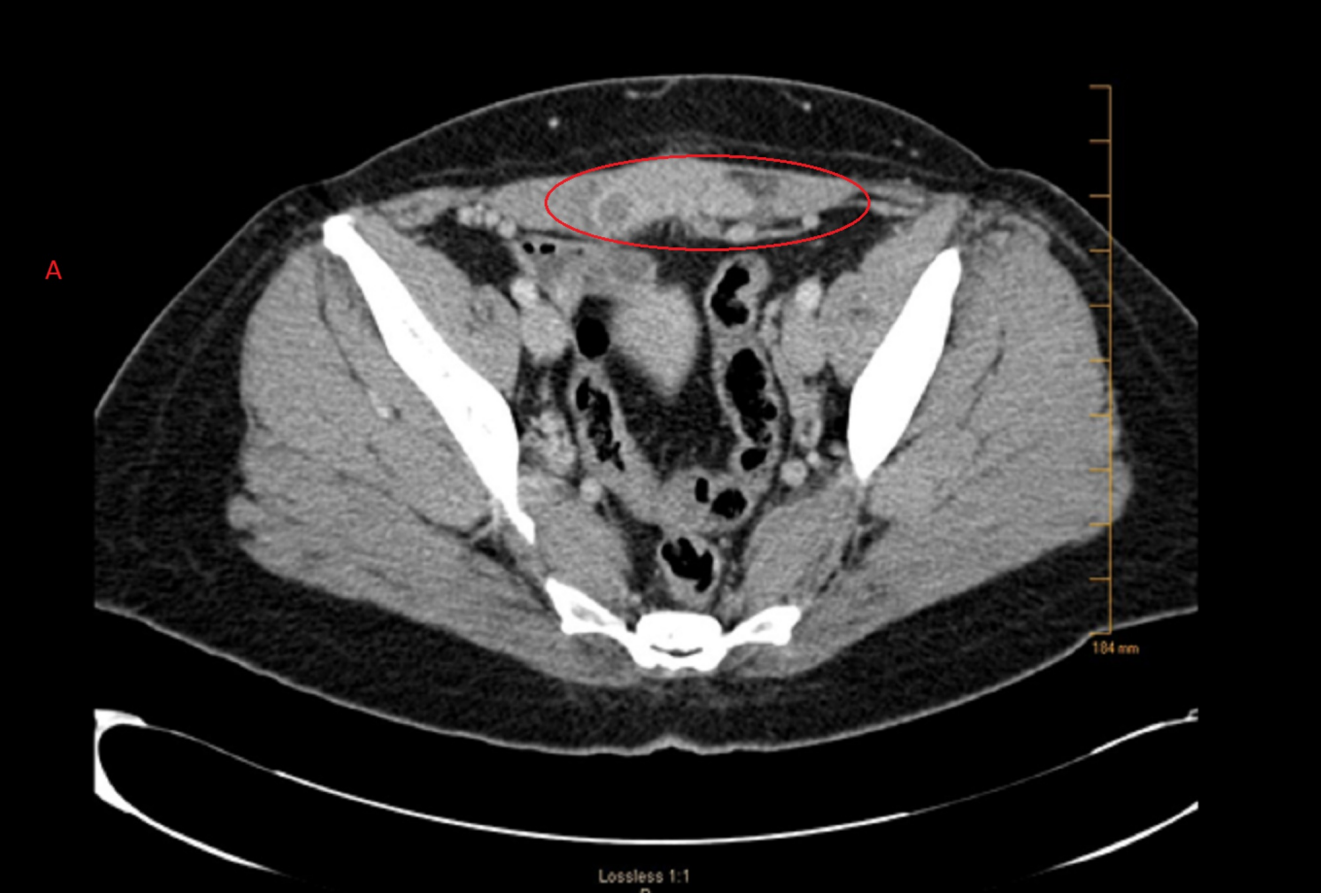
At 12-month follow-up, patient was two months off hormonal therapy, and axial computed tomography scan revealed abdominal mass remained shrunken (oval ring)
